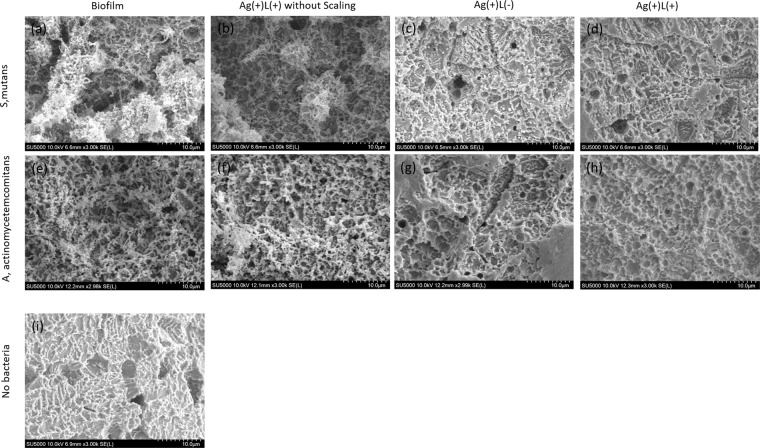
SEM images of the condition of the titanium surface. (a) Infected by *S. mutans* for 48 h. (b) Infected by *S. mutans* followed by Ag(+)L(+) treatment without ultrasound scaling. (c) Infected by *S. mutans* followed by Ag(+)L(−) treatment after ultrasound scaling. (d) Infected by *S. mutans* followed by Ag(+)L(+) treatment after ultrasound scaling. (e) Infected by *A. actinomycetemcomitans* for 48 h. (f) Infected by *A. actinomycetemcomitans* followed by Ag(+)L(−) treatment without ultrasound scaling. (g) Infected by *A. actinomycetemcomitans* followed by Ag(+)L(−) treatment after ultrasound scaling. (h) infected by *A. actinomycetemcomitans* followed by Ag(+)L(+) treatment after ultrasound scaling. (i) Sandblasted and acid-etched with no infection by bacteria.
